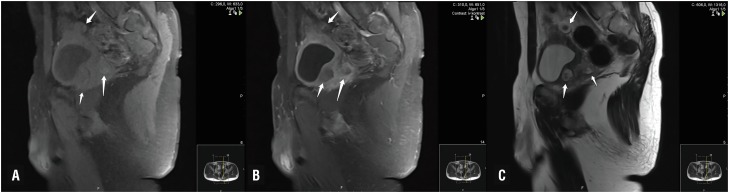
MRI sagittal images; nodular lesions marked with arrows A. T1 weighted fat suppressed B. Post contrast T1 weighted fat suppressed C. T2 weighted image.
